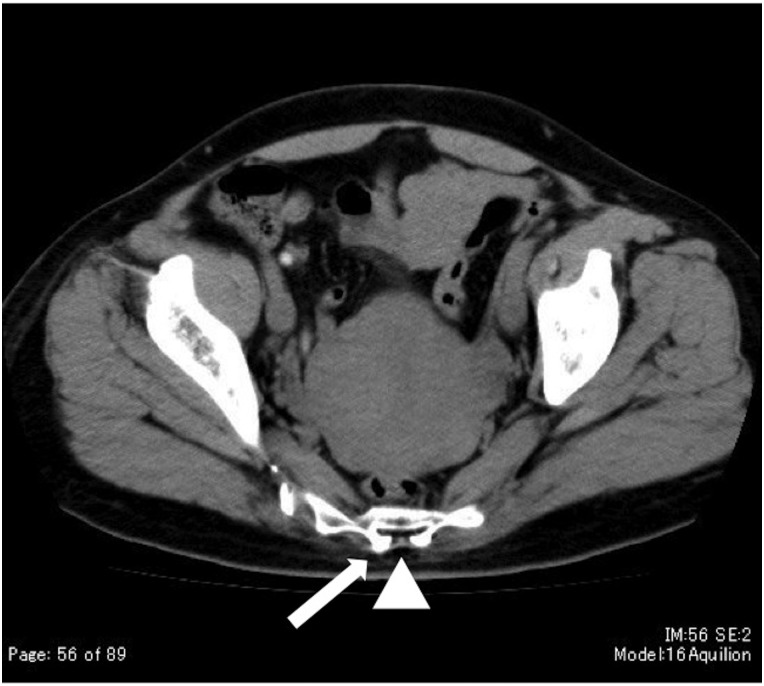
CT image of a 51-year-old woman. Arrowhead: sacral hiatus. Arrow: sacral cornua.
